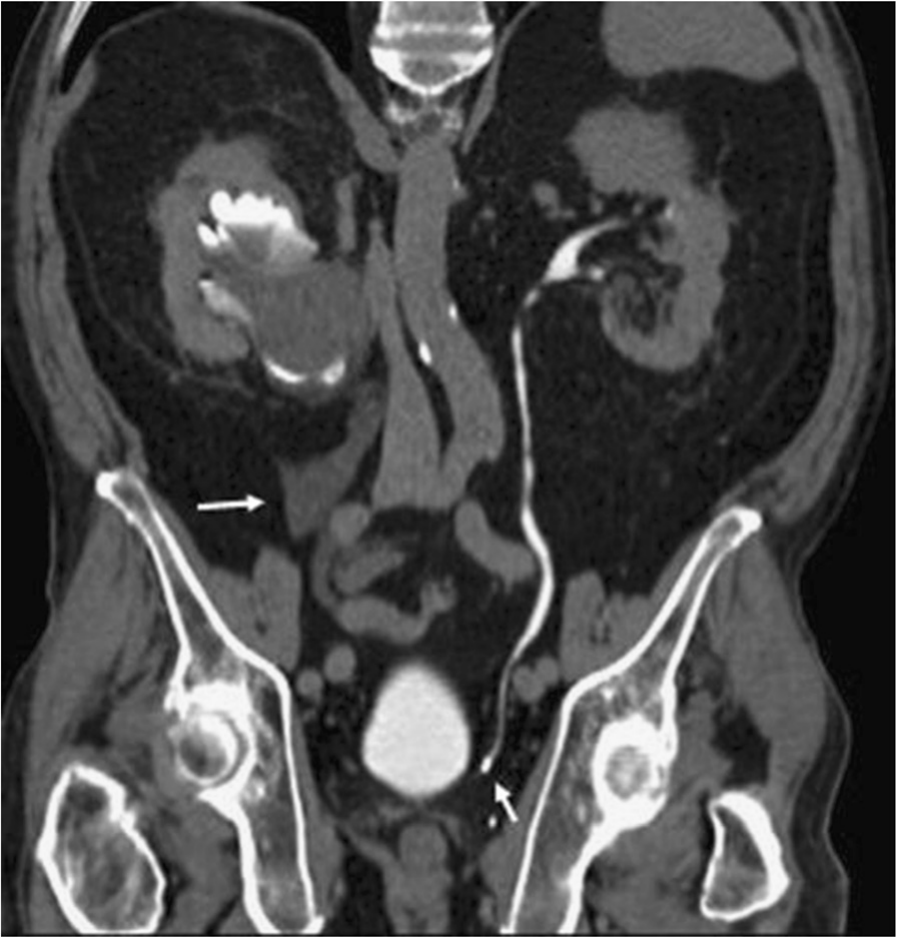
Coronal maximum intension projection reconstruction from excretory phase of the current computed tomography scan. This image shows the cyst with contrast inside, free fluid on the retroperitoneal space (big arrow) and the stone obstructing the left ureter (small arrow).
